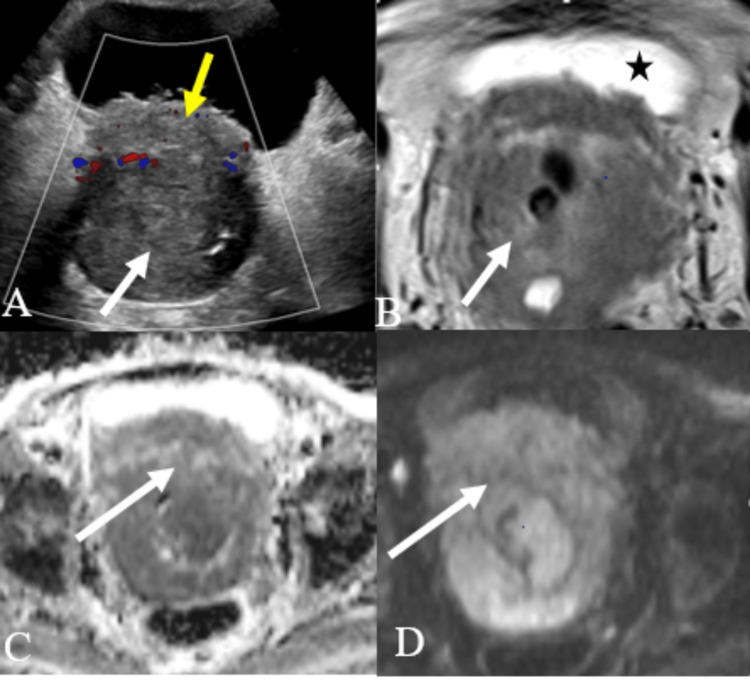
A case of cervical carcinoma A: USG color Doppler image of the pelvis showing bulky cervix with a heterogeneously hypoechoic lesion (white arrow), demonstrating internal vascularity. The lesion is seen infiltrating the posterior wall of the bladder (yellow arrow). B: Axial T2 pelvis MR image showing a large, fairly defined heterogeneously hyperintense lesion (white arrow) in the cervix. The lesion is seen infiltrating the posterior wall of the urinary bladder (black star). C and D: Axial section of DWI MRI of the pelvis and corresponding ADC where the lesion is showing patchy areas of restriction of diffusion (white arrow), suggestive of cervical malignancy. USG - ultrasonography; DWI - diffusion-weighted imaging; ADC - apparent diffusion coefficient
